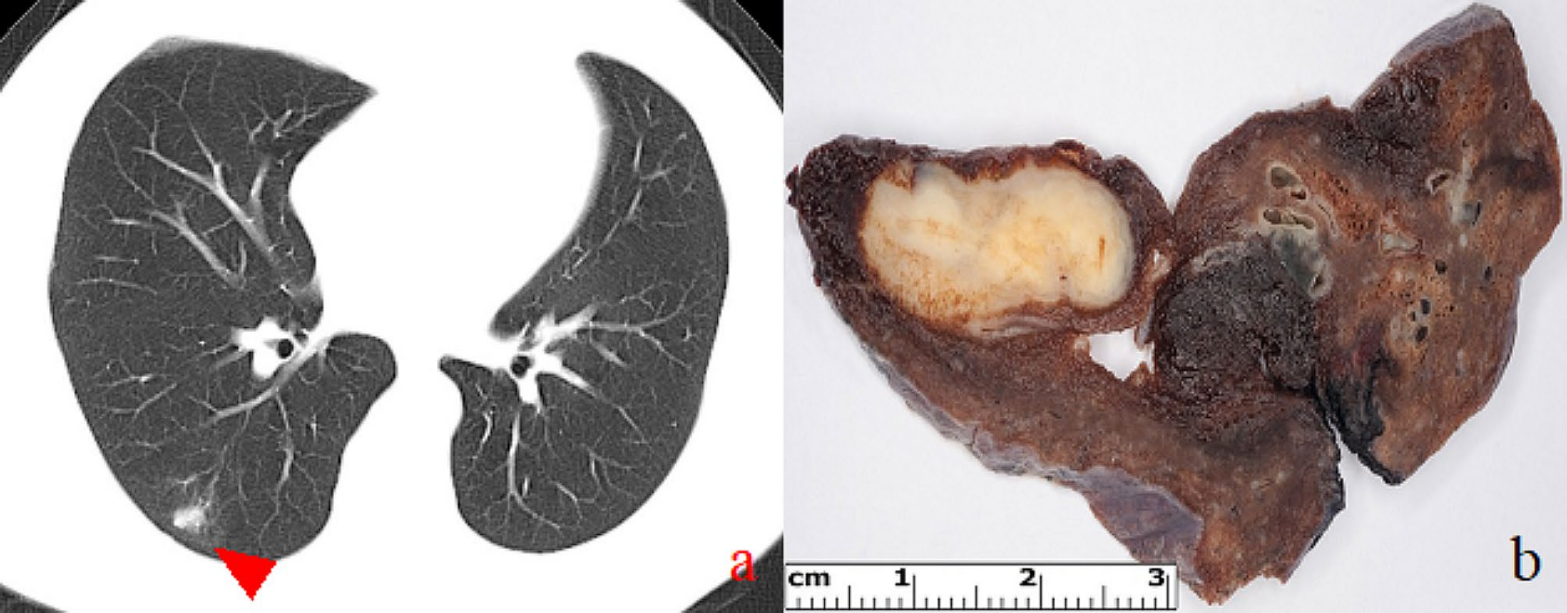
Radiological and macroscopic appearance of human pulmonary dirofilariasis. (a) On the chest CT scan, a peripheral coin lesion was observed in the lung parenchyma (red arrowhead). Otherwise, the CT scan found no abnormalities in the thorax. (b) Grossly, a well-defined, yellow-white nodule was situated in the subpleural area. Additionally, focal bleedings were present in the lung parenchyma
